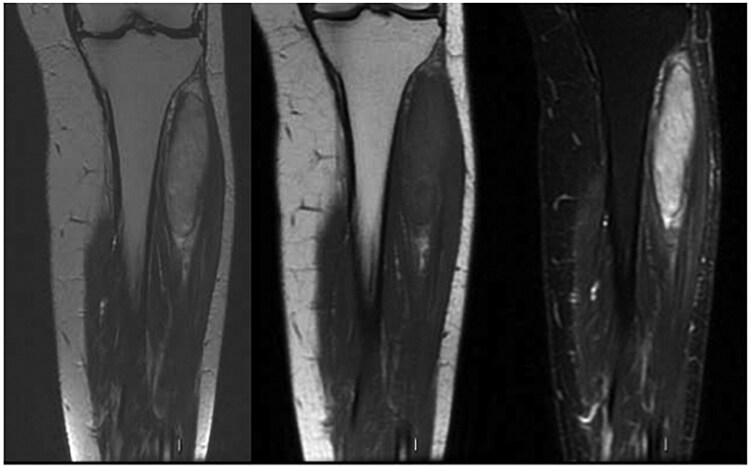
MRI after radiotherapy. (A) Coronal T2-weighted image; (B) Coronal T1-weighted image; (C) Coronal STIR image. Significant volumetric reduction of the liposarcomatous mass is evident.
